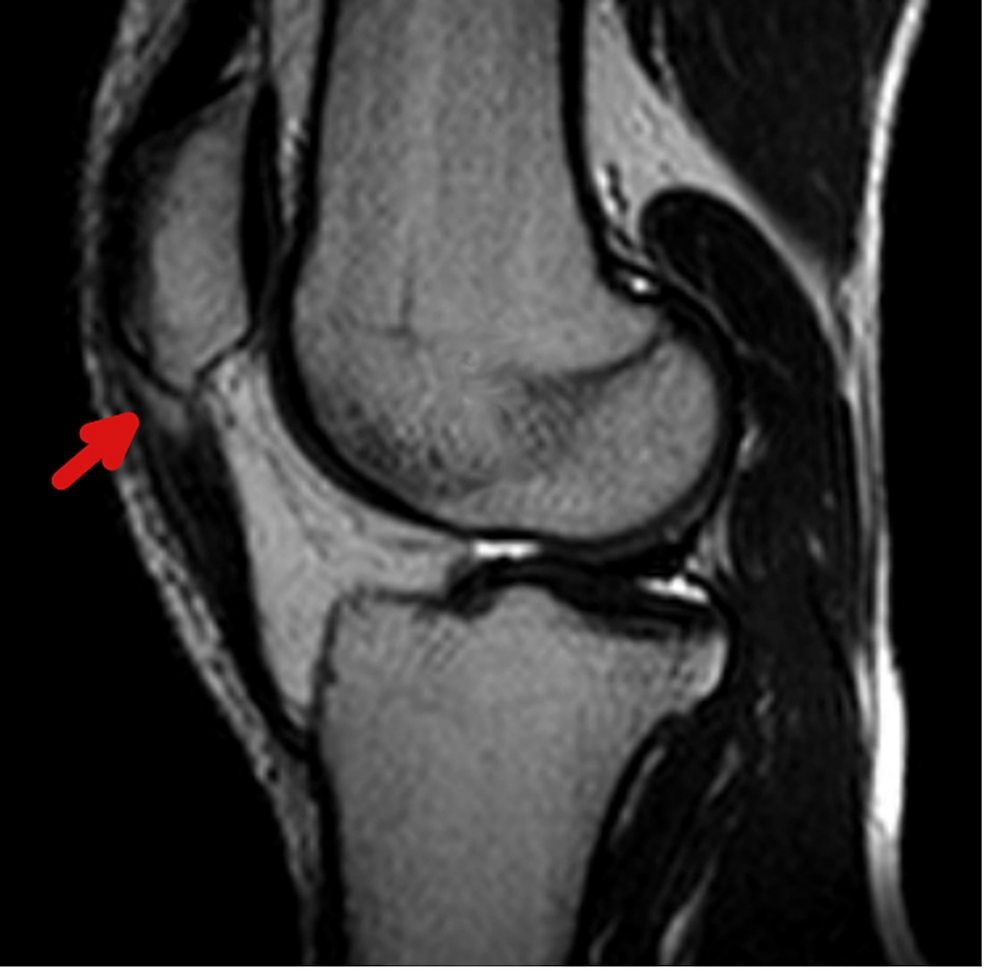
Sagittal T2-weighted MRI of the right knee of the first patient The nidus of tendinopathy is plainly visible just distal to the inferior patellar pole (arrow) MRI: magnetic resonance imaging
